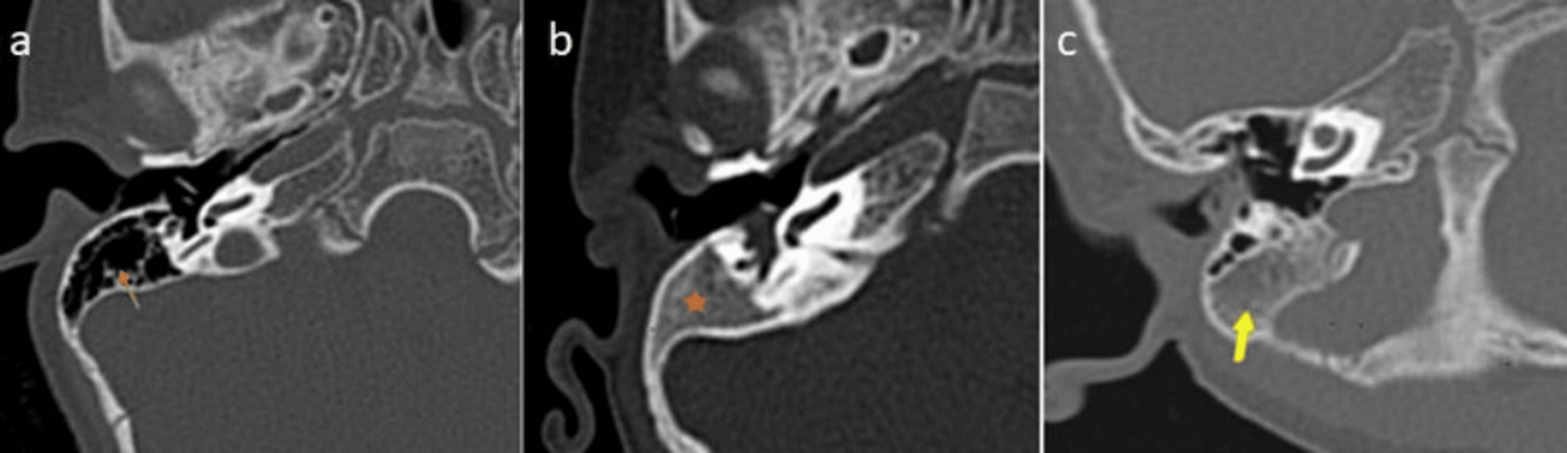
(a-c) Mastoid pneumatization HRCT of the temporal bone showing (a) a well-pneumatized mastoid (arrow), (b) a non-pneumatized right mastoid (star), and (c) few mastoid air cells indicating a hypopneumatized mastoid. HRCT: high-resolution CT
